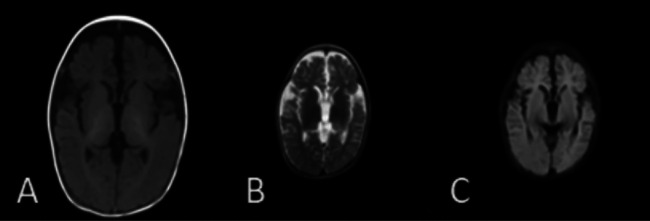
Brain MRI of the younger brother [(A) T1, (B) T2, (C) T2 FLAIR]: brain MRI of the younger brother revealing a widening of cerebral sulci and fissures, cortical thinning, and enlargement of the frontal and temporal angles at the age of 14 months.
